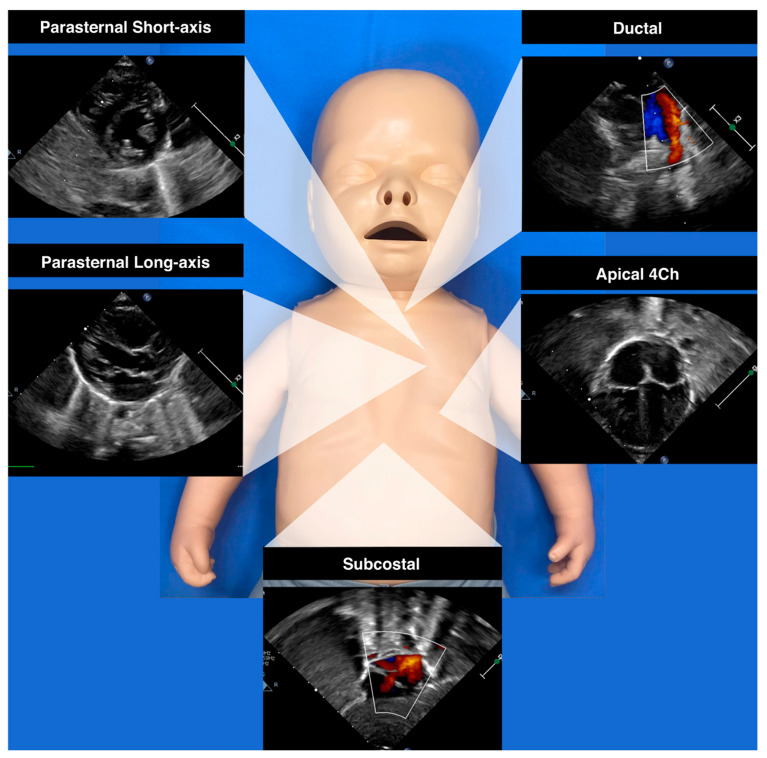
Standard echocardiographic views used in NPE.
